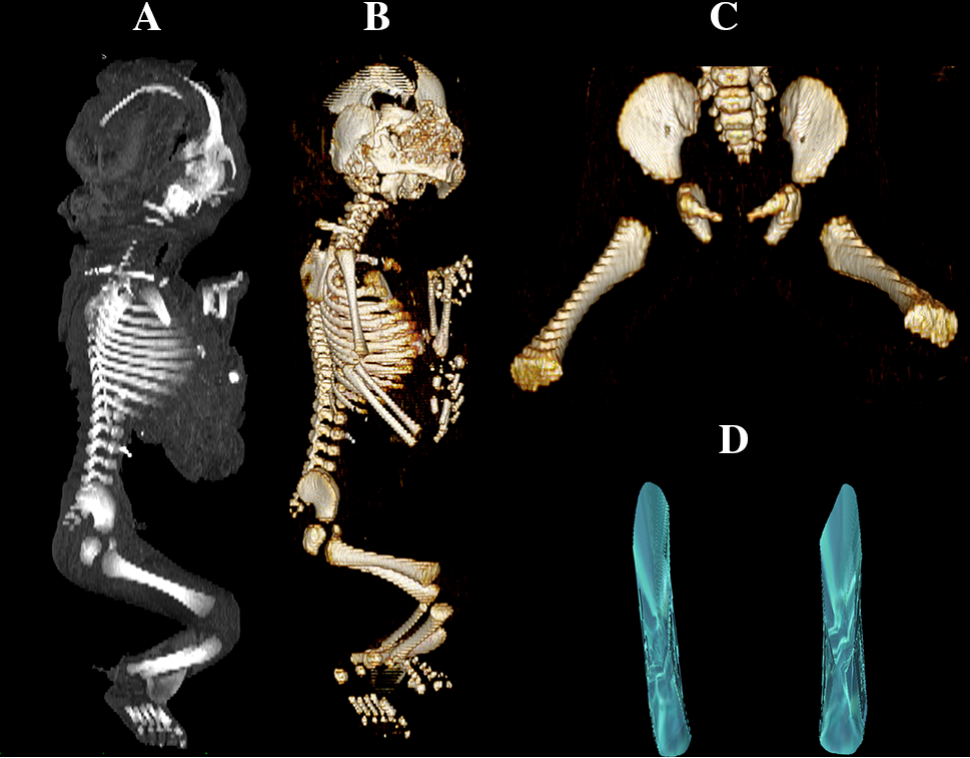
CT of a female fetus aged 27 weeks (in the sagittal projection) recorded in DICOM formats (a), with the sagittal reconstruction of its bones (b), with the frontal projection of its pelvic girdle and right and left femurs (c), and with 3D reconstructions of its right and left femoral shaft ossification centers (d) assessed by Osirix 3.9
